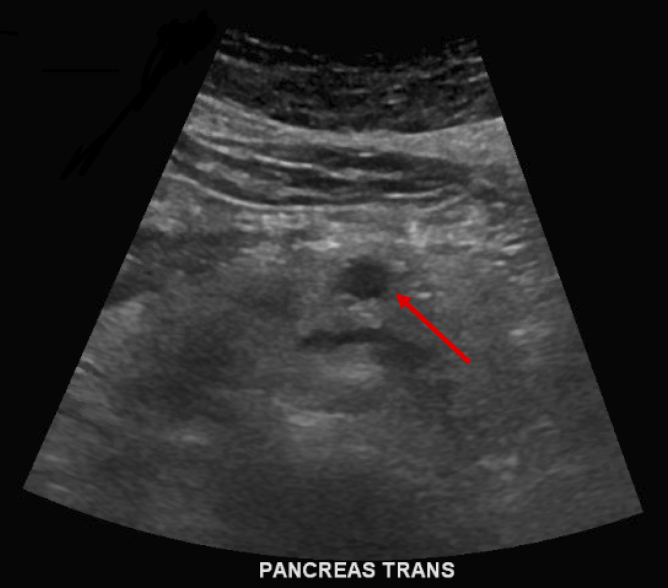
Transabdominal ultrasound demonstrating a well-circumscribed hypoechoic pancreatic lesion without pancreatic ductal dilation. Red arrow denotes the lesion.
